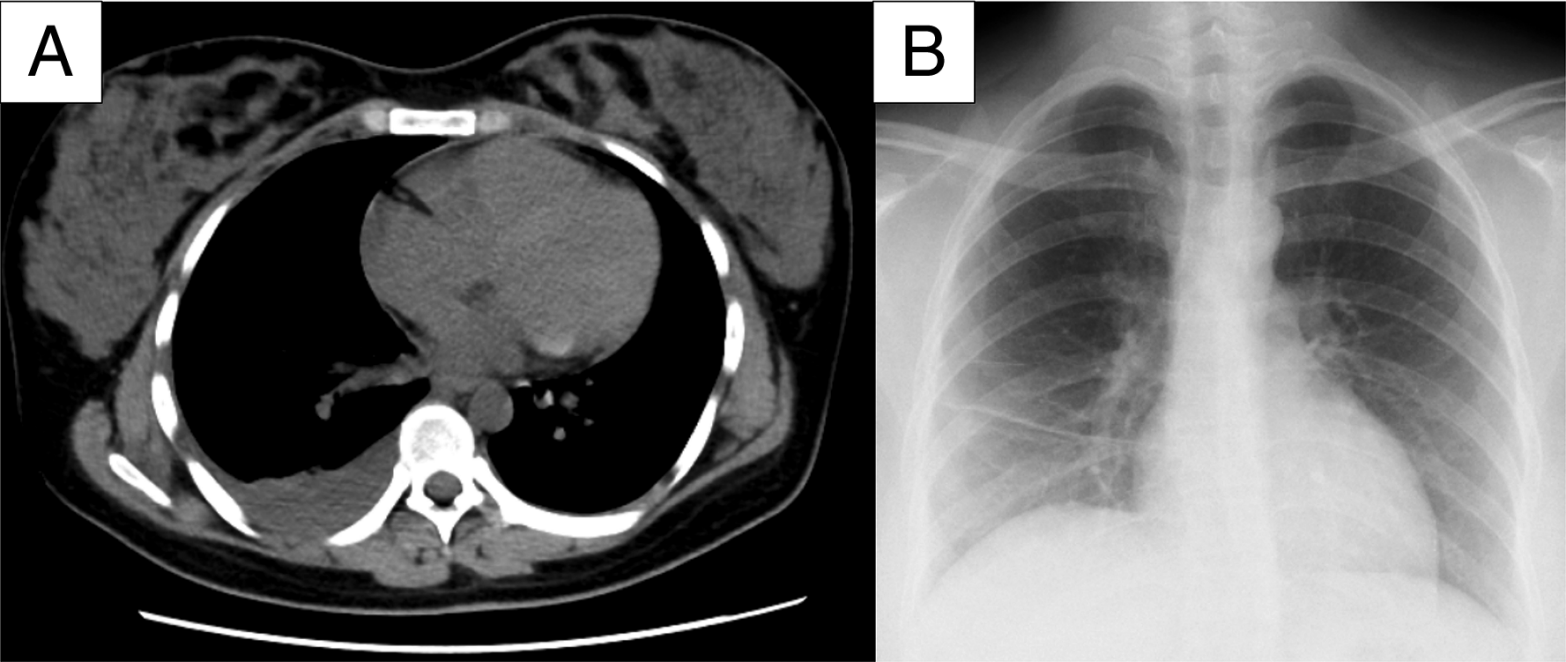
(A) chest computed tomography on postoperative day (POD) 7 demonstrated a right‐sided pleural effusion without loculations; (B) chest radiograph on POD 50 only showed a linear atelectasis in the right lower lung field.
